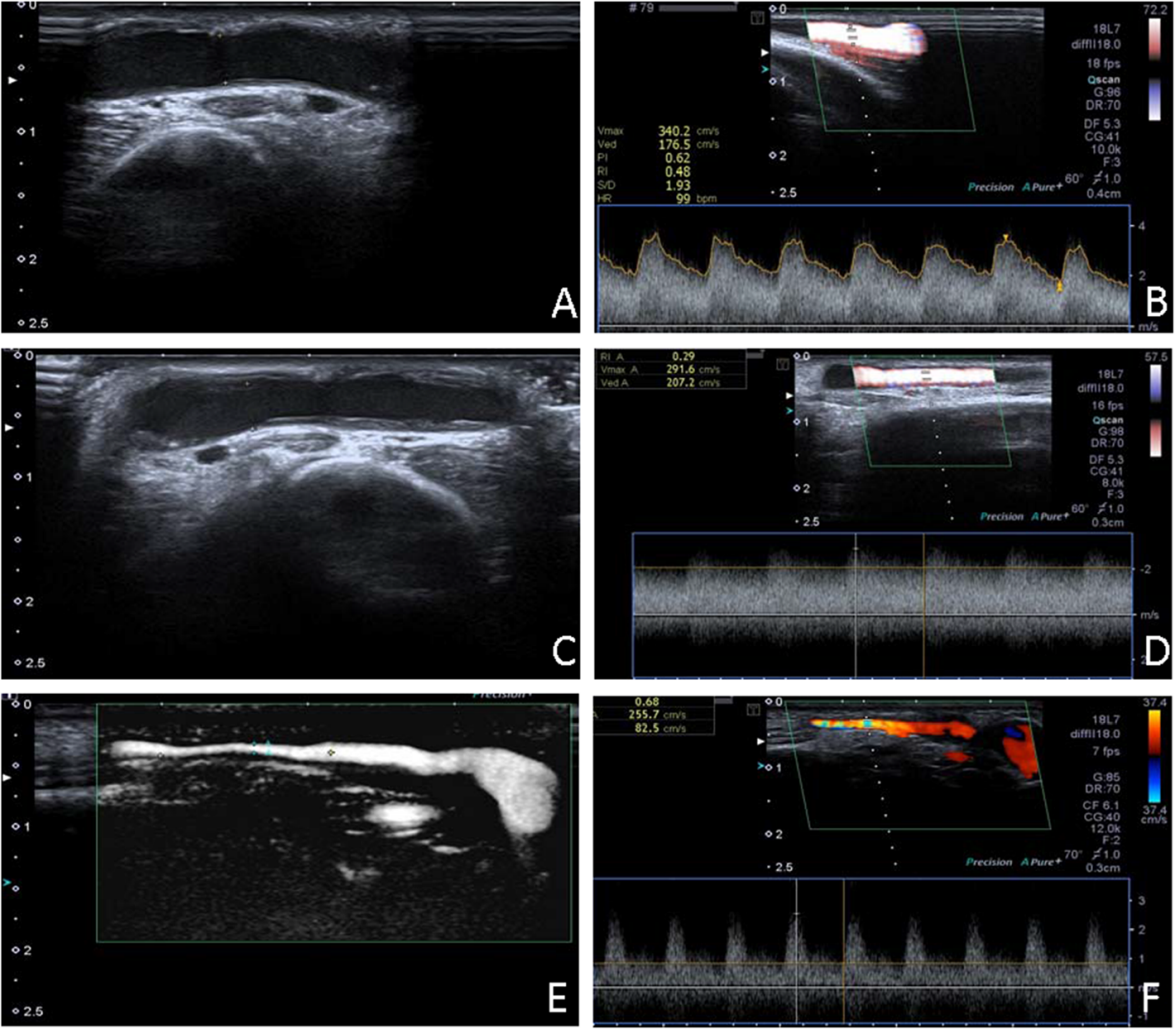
Vascular ultrasound follow-up after the endovascular treatment. At the 2-, 6-, and 18-month follow-ups, the anastomose site diameter and AVF blood flow were 3.8 mm (a) and 340 cm/s (b), 3.8 mm (c) and 291 cm/s (d), and 0.8 mm (e) and 255 cm/s (f), respectively
